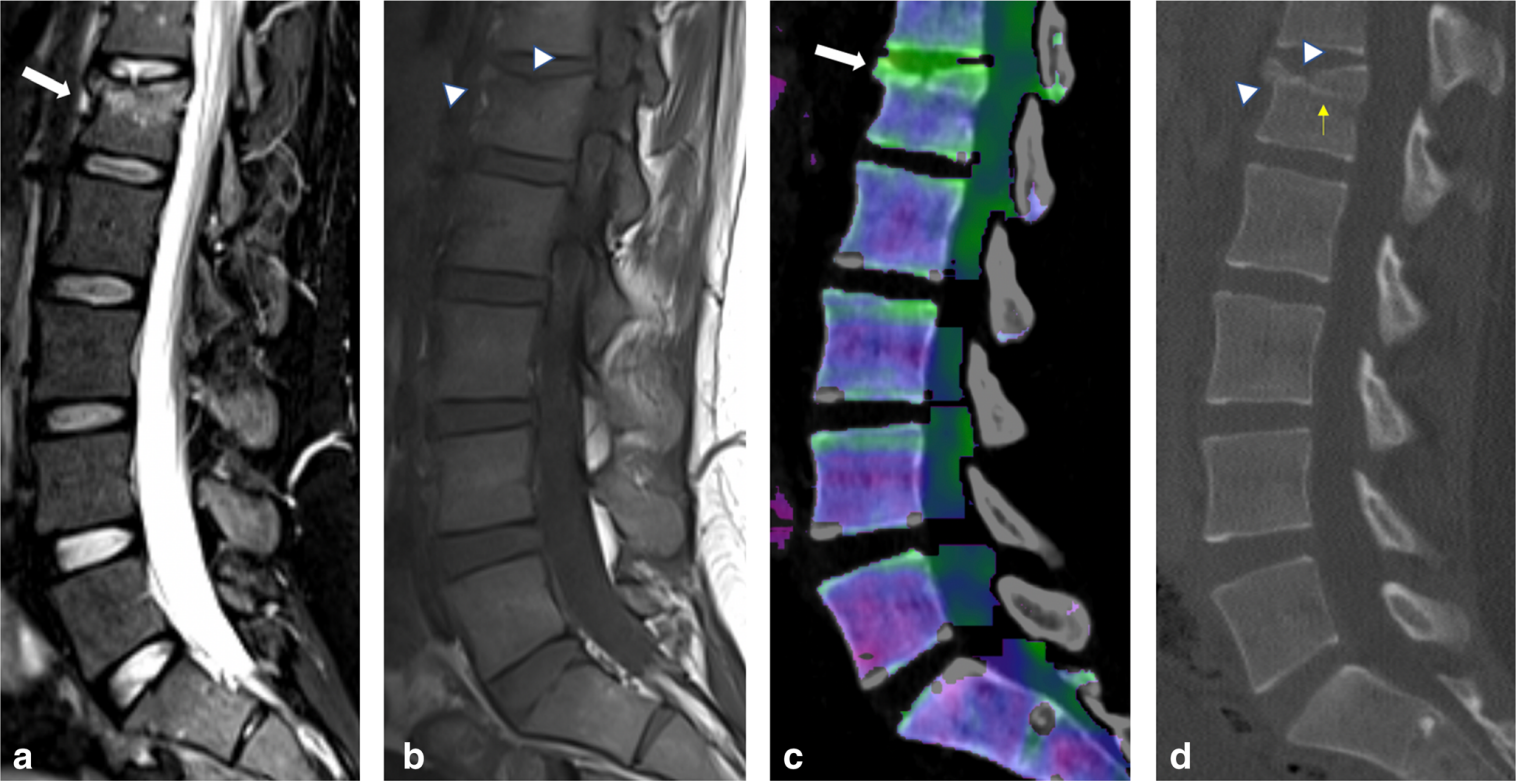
Twenty-six-year-old woman presenting with spinal trauma after motorcycle accident. a Sagittal turbo inversion recovery magnitude (TIRM)–magnetic resonance imaging (MRI) series showing bone marrow edema (BME) in the upper two quadrants of L1 (*arrow*). b Sagittal spin-echo (SE) T1-weighted MRI demonstrating two distinct acute fracture lines affecting the anterior and upper (*arrowheads*) cortical surfaces. c Dual-energy computed tomography (DECT)–virtual non-calcium (VNCa) images depicting BME in the two upper quadrants (*arrow*, displayed as green area). There was a complete agreement between DECT and MRI concerning presence, extent (2 quadrants) and diagnostic confidence (score, 3) regarding BME detection. d Sagittal grayscale DECT series not only showing the two cortical fracture lines (*arrowheads*), but also a horizontal hyperdense line indicating trabecular impaction (*yellow arrow*), detected and interpreted as posterior edge involvement by all readers, which was missed on sagittal T1-weighted MRI series by each reader. Confidence in depicting fracture lines was rated as high (score 3) by 5/5 readers on both MRI and DECT series
